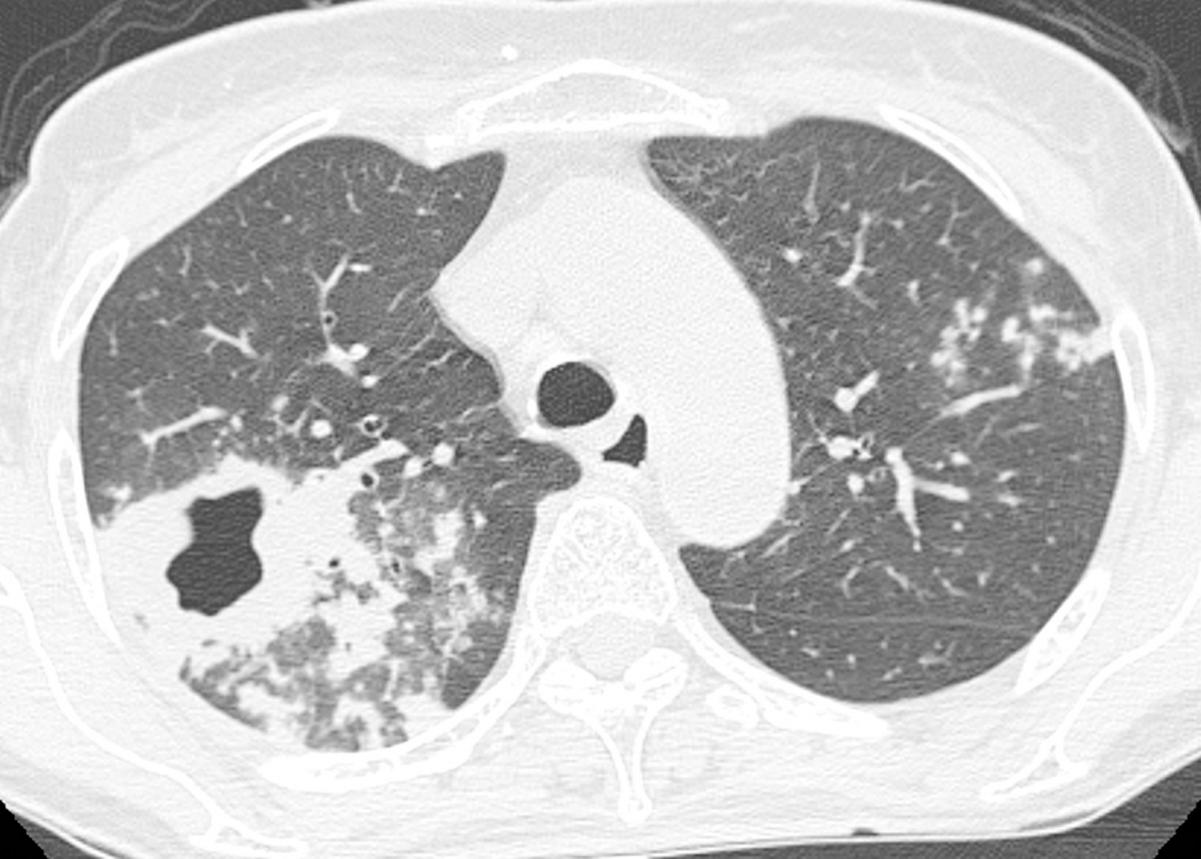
Primary multidrug-resistant tuberculosis with 37-year-old woman. The thin section CT (1mm slice thickness) with high-spatial-frequency algorithm and lung window setting shows thick wall cavity and subsegmental or lobular consolidation in right upper lobe. Tree-in-bud lesion is seen in left upper lobe.
